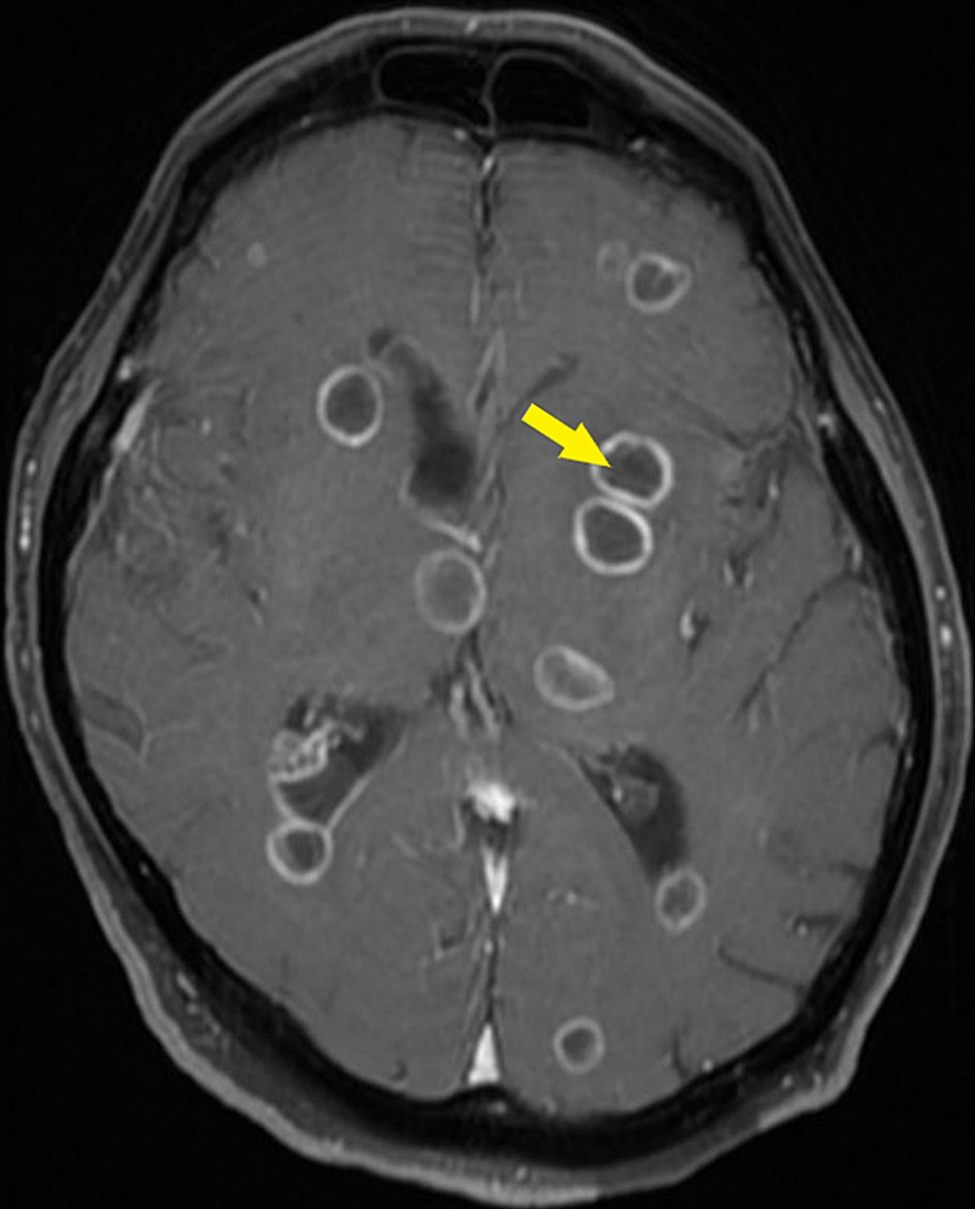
Magnetic resonance imaging showing innumerable parenchymal brain abscesses
